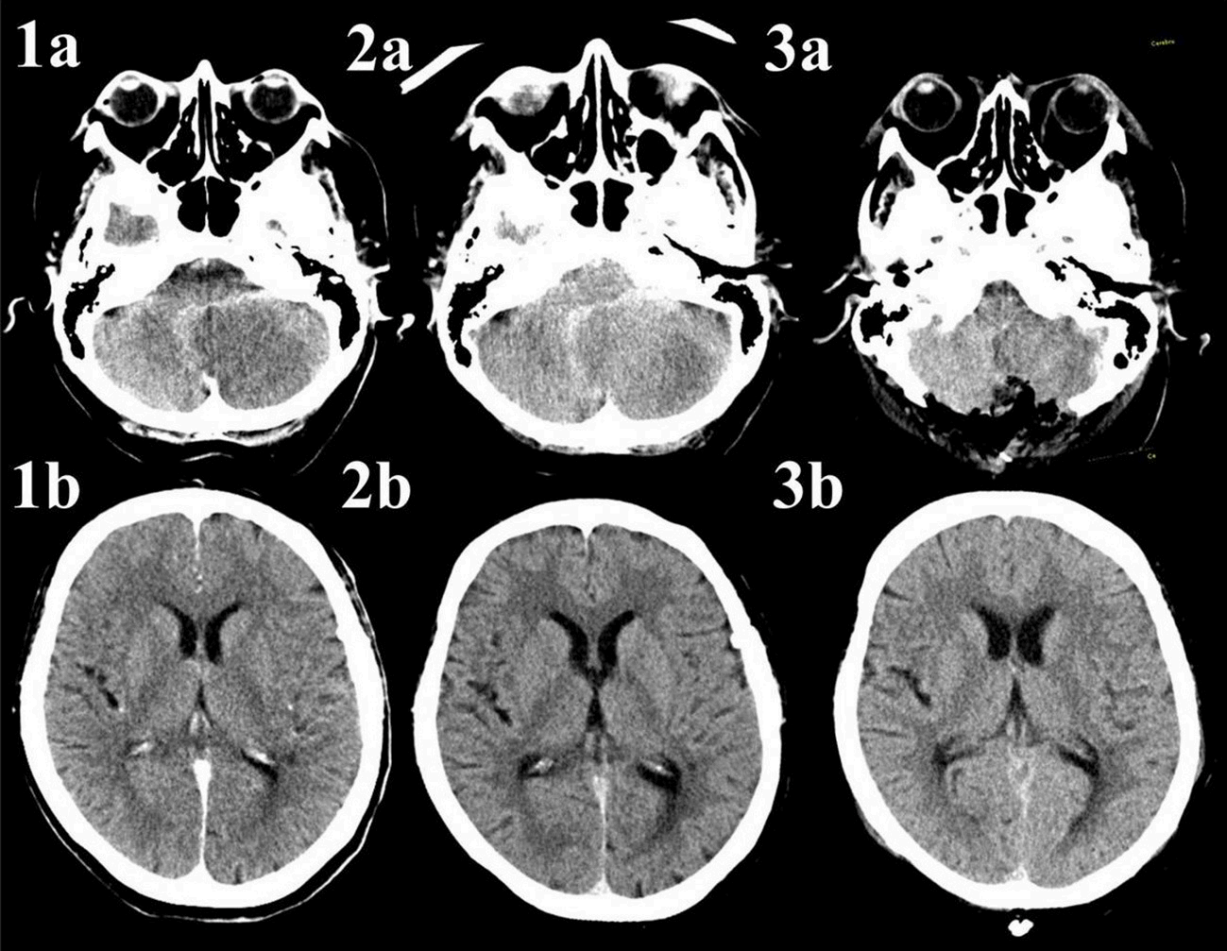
Native CT-scans of a patient with infarction of the left posterior inferior cerebellar artery. The patient underwent no acute treatment due to late arrival and already visible ischemic changes. Image 1a shows the hypodense area on the left side of the cerebellum, there are no signs of cerebrospinal fluid circulation disturbance on Image 1b. Image 2a reveals progressive infratentorial edema with resulting enlargement on the ventricular system due to compression of the fourth ventricle (Figure 2b). Images 3a,b present a scan 1 day after suboccipital craniectomy. Whilst there is still enlargement of the frontal horns of both lateral ventricles, the third ventricle is slightly smaller, indicating flow restoration. The extraventricular drainage (EVD), implanted during the decompressive craniectomy, is not shown in these images.
